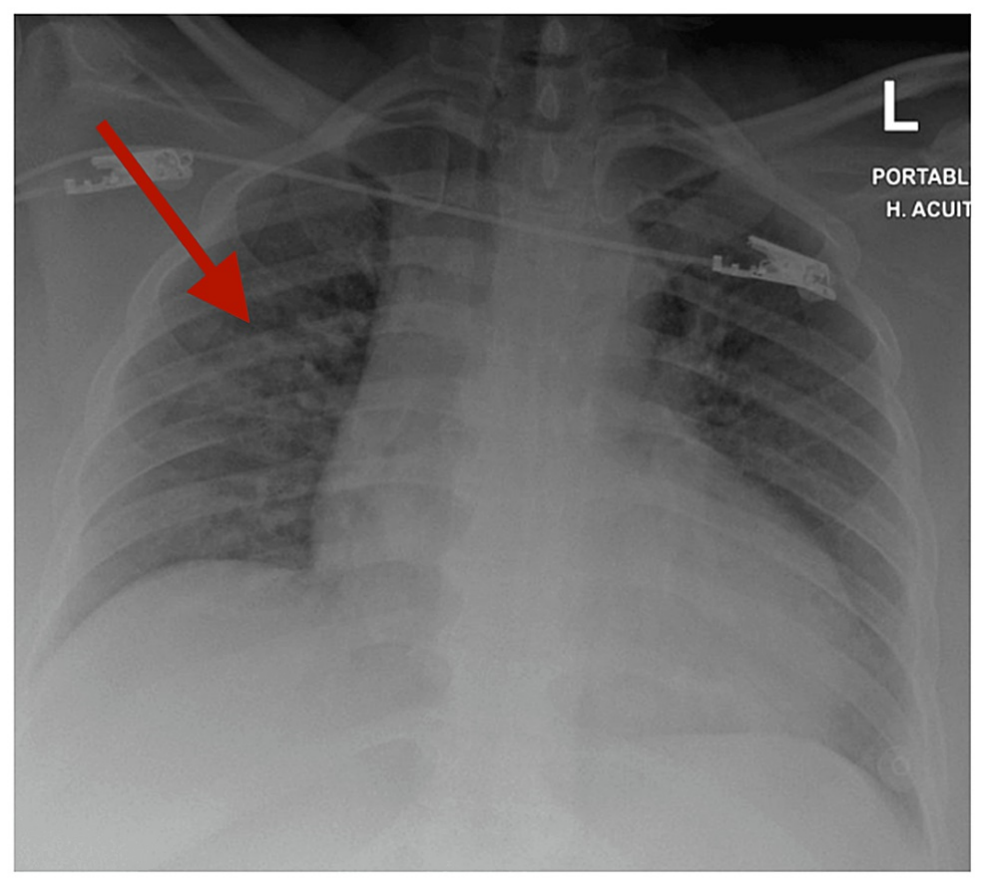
Chest x-ray (prominent broncho vascular markings with focal infiltrates in the right middle zone)
